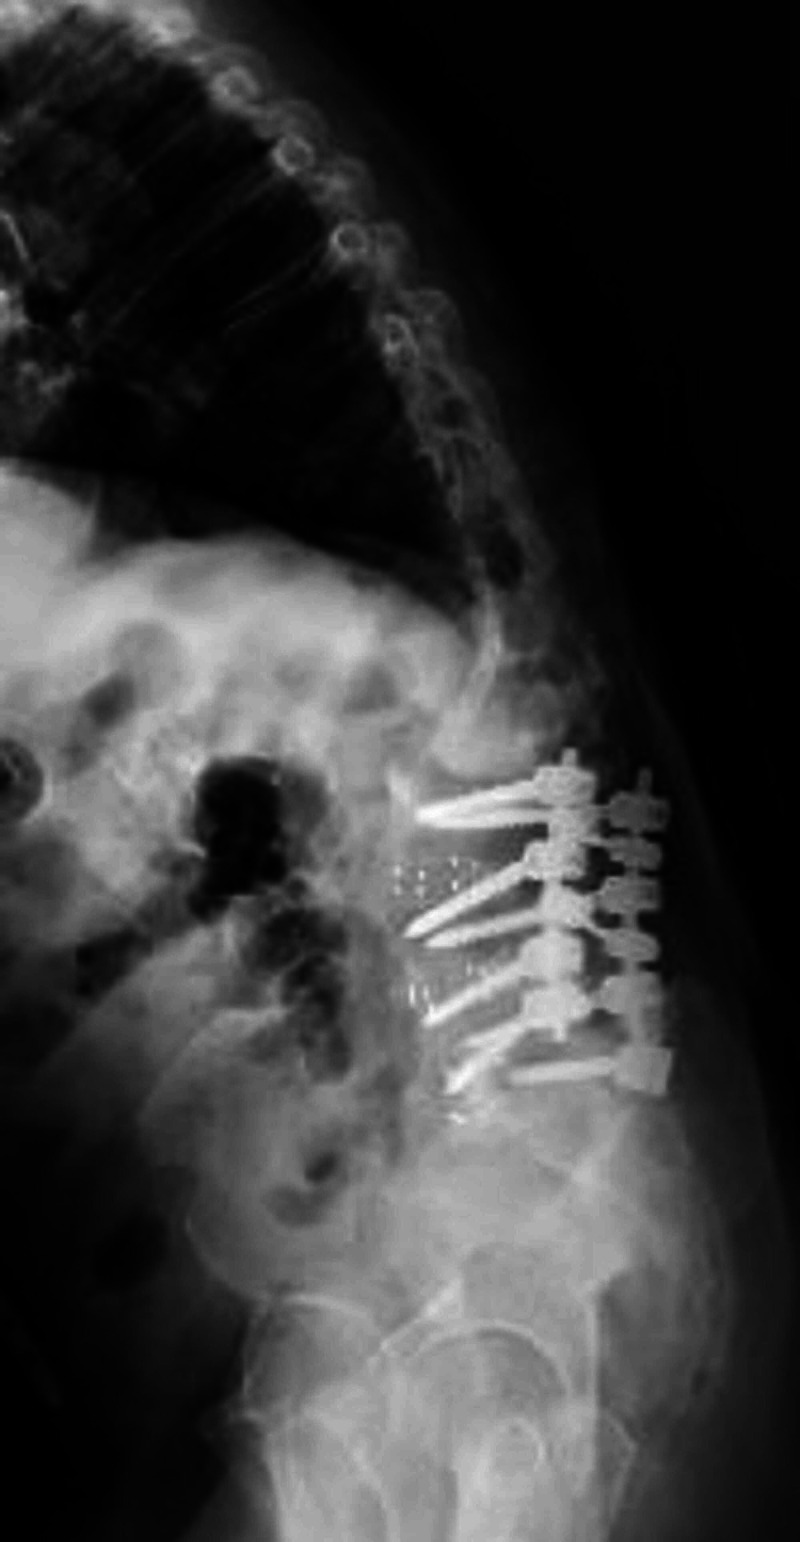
Preoperative standing upright scoliosis x-ray films shows the kyphotic deformity with patient’s inability to stand upright prior to kickstand rod surgery.
